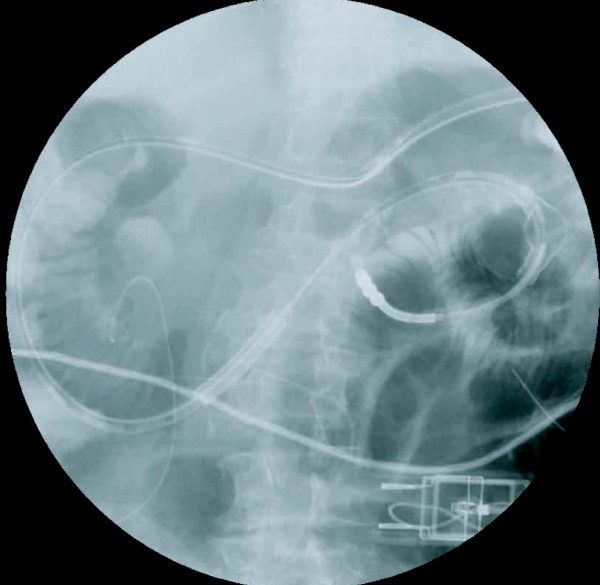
Abdominal radiograph demonstrating percutaneous gastrojejunostomy tube placement. The apex of the gastrojejunostomy tube was introduced into the upper jejunum 1100 mm from the insertion point. Severe intestinal distention can be seen.
